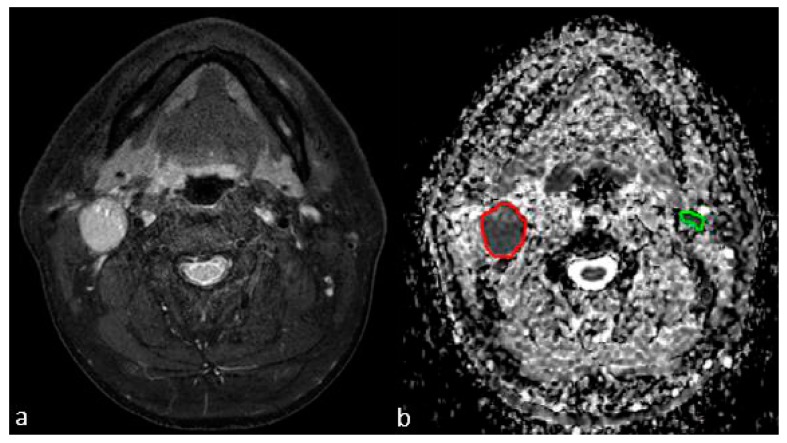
Images in a 54-year-old man with right tonsillar squamous cell carcinoma. (a) An enlarged lymph node (LN) larger than 1 cm is seen in right neck level IIA and another subcentimeter-sized LN is seen in left neck level IIA on the axial fat-suppressed T2-weighted image. (b) Region-of-interest is drawn over the enlarged right level II LN (red) and the left level II subcentimeter-sized LN (green) on the apparent diffusion coefficient (ADC) map, acquired in the axial plane using 2D navigated interleaved multi-shot echo-planar imaging-based diffusion-weighted imaging (msEPI-DWI).
